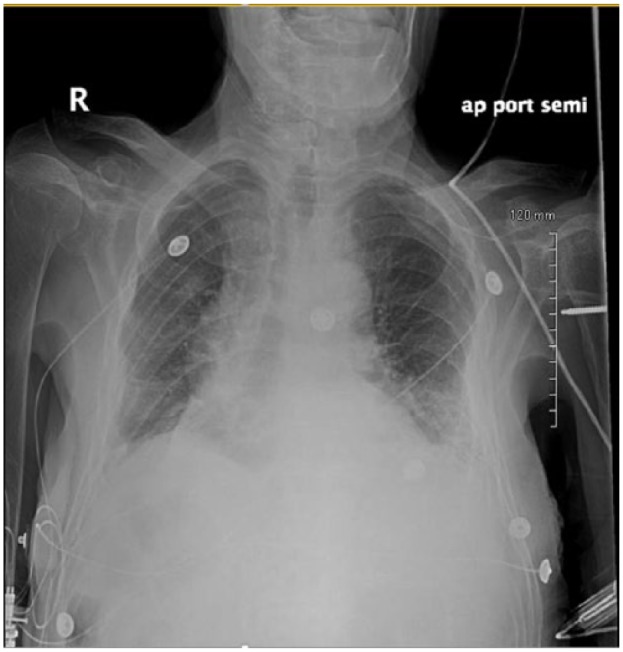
Chest X-ray showing bilateral bibasilar infiltrates with subtle bilateral pleural effusion more on the right side.
